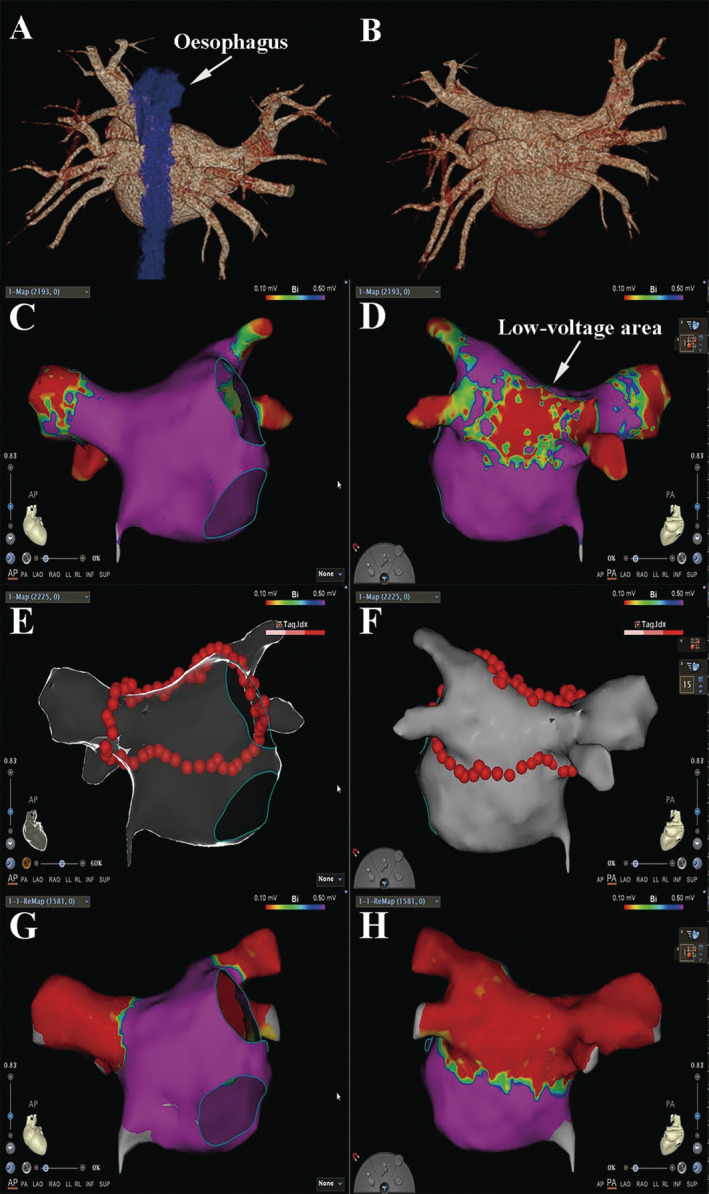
Preoperative left atrial computed tomography angiography revealed a common ostium of the bilateral inferior pulmonary veins into the left atrium, with the common ostium being close to the esophagus (A, B). Intraoperatively, mapping confirmed predominantly uniform high‐voltage in most areas of the left atrium (C), with only the posterior wall exhibiting a low‐voltage area (D). During catheter ablation, a single‐ring ablation strategy was employed (E: Anterior–posterior position, F: Posterior–anterior position). Post‐catheter ablation, successful isolation of both pulmonary veins and the low‐voltage area in the left atrial posterior wall was achieved (G: Anterior–posterior position, H: Posterior–anterior position).
